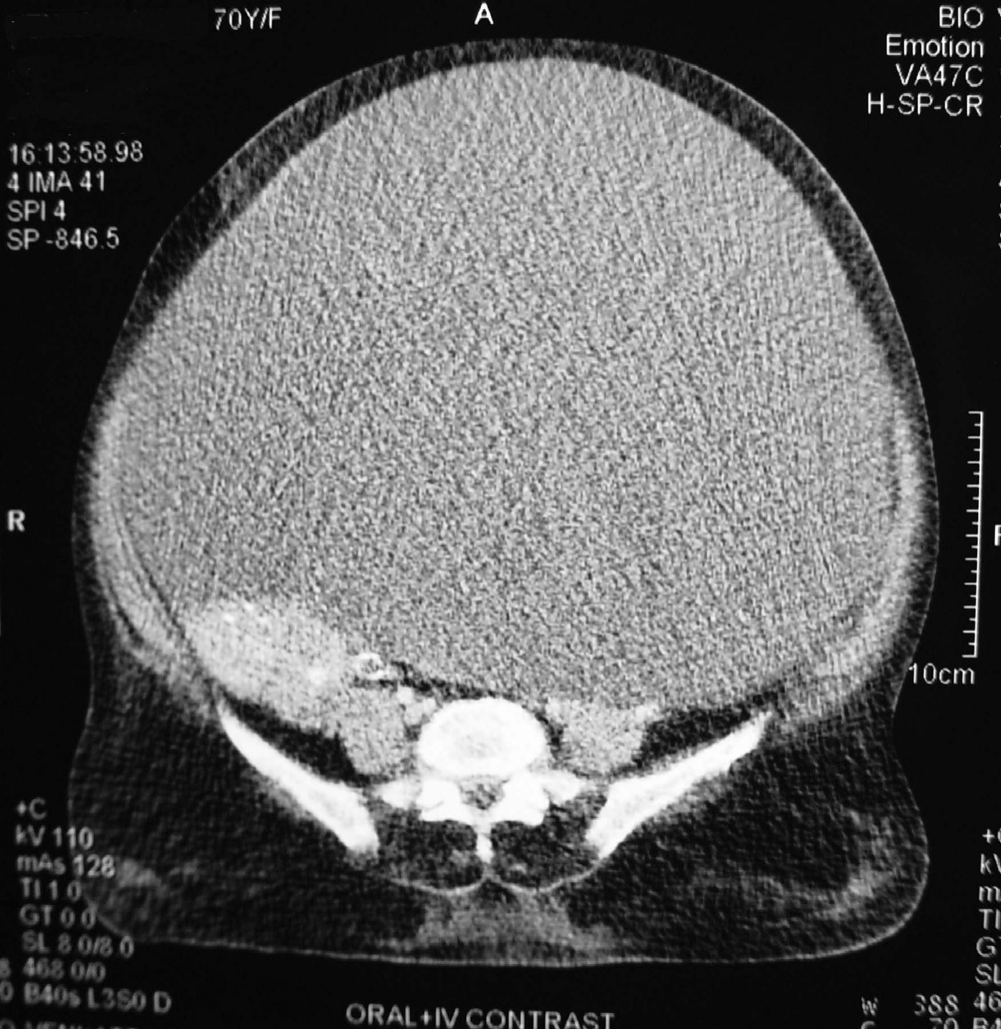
Computed tomography scan of the abdomen and pelvis showing a large cystic mass occupying the entire abdomen and pelvis.
